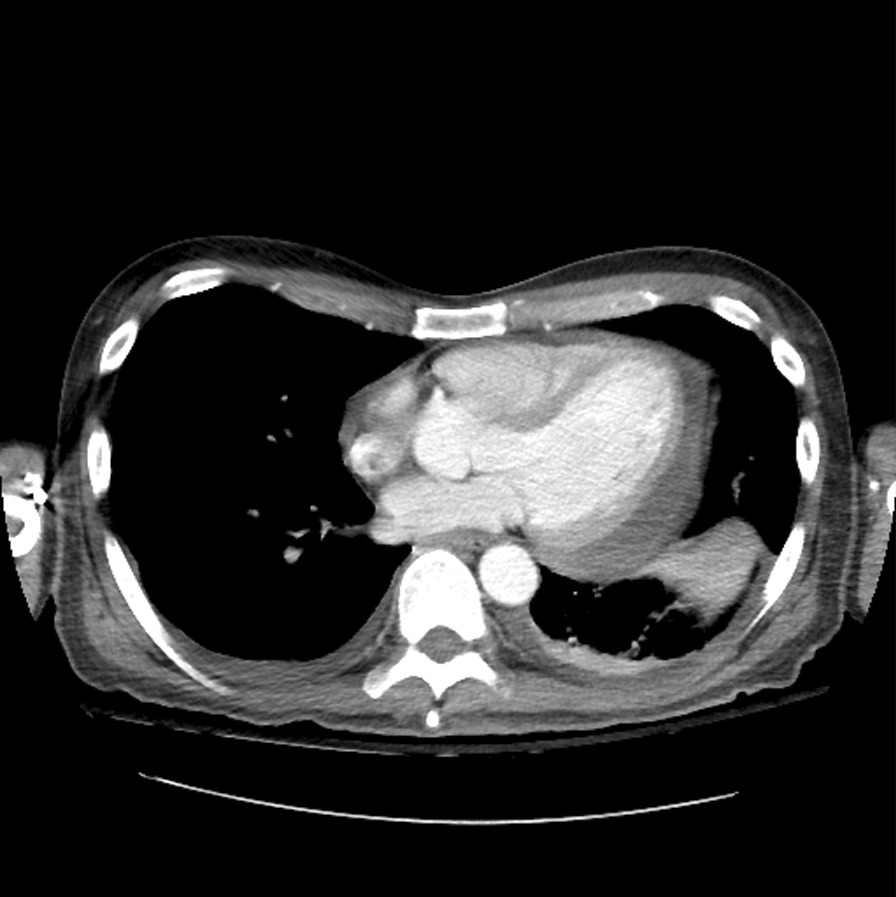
51 year-old male with chronic presenting with shortness of breath. Axial CT image at the level of the heart demonstrates a small pericardial effusion with thickening/enhancement, trace pleural effusions and left basilar consolidation
